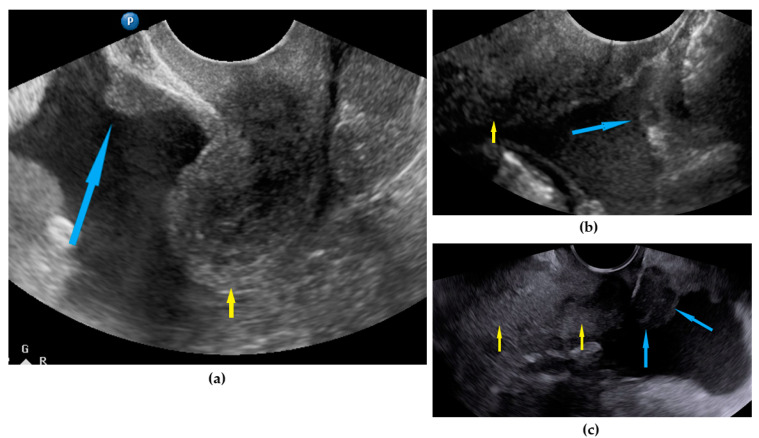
Ultrasound images of pelvic peritoneum involvement: (a) nodule on bladder peritoneum; nodule (b) and tumor (c) on pouch of Douglas peritoneum. Arrows: blue identify nodules, yellow identify uterus.
